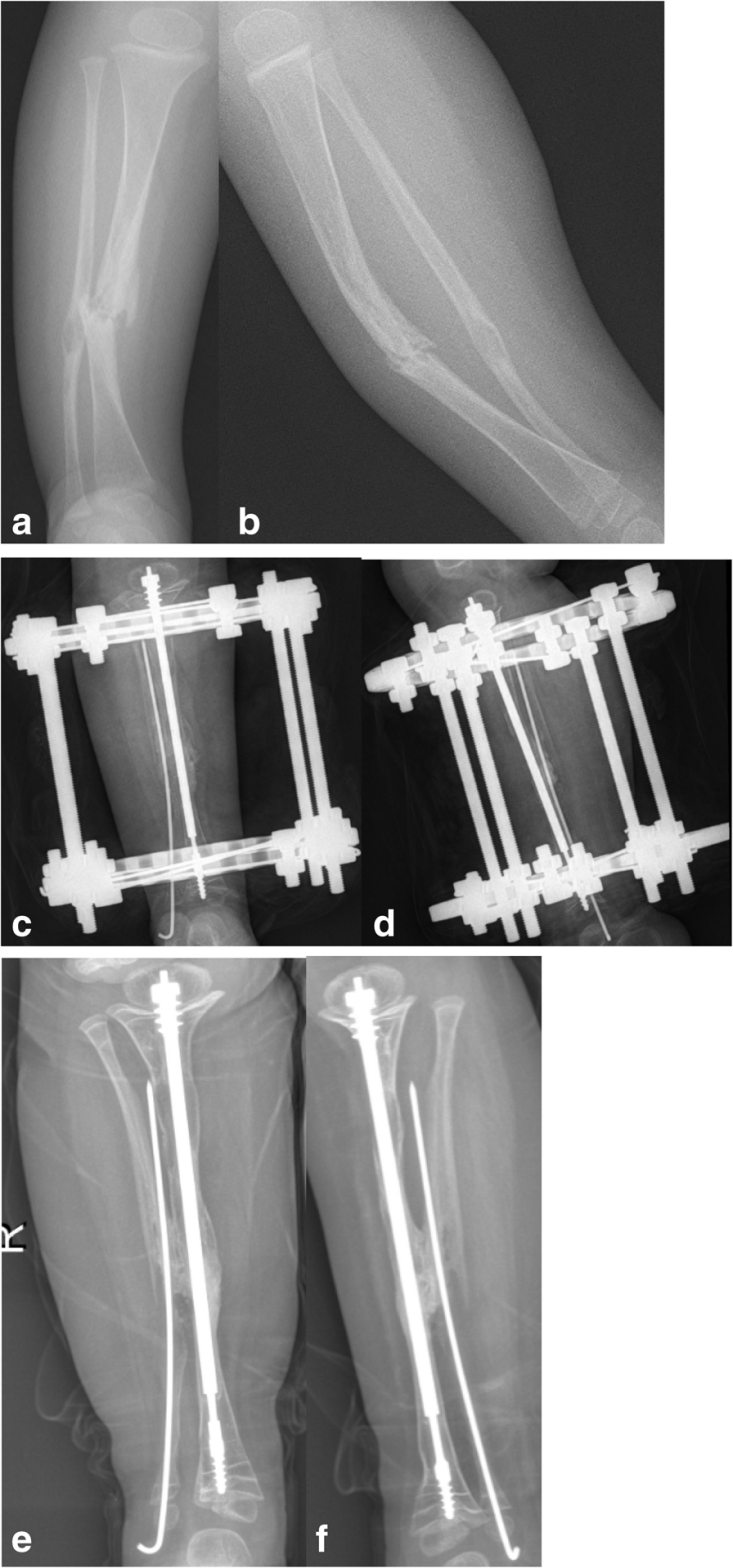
a, b A 2-year-old boy. Preoperative anteroposterior and lateral radiographs. c, d Four months post-operation. e, f Seven months post-operation with telescopic rod displacement and epiphyseal plate tethering
